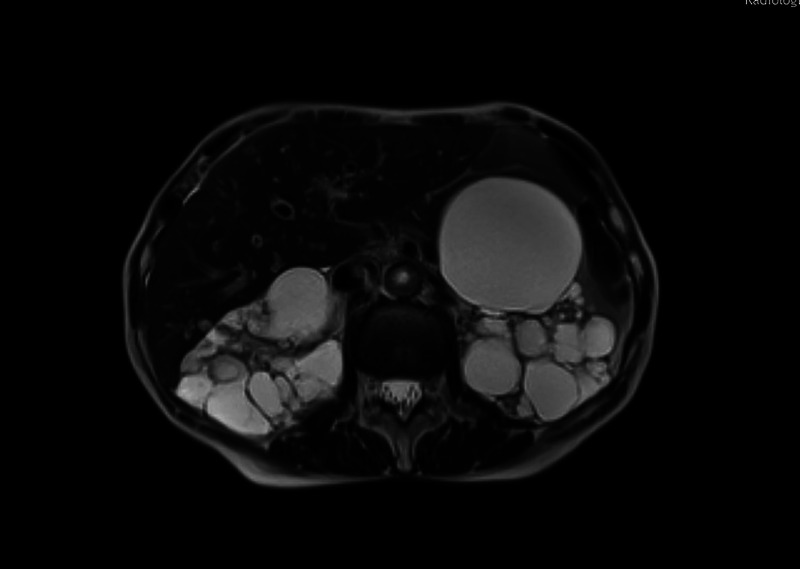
Initial magnetic resonance imaging demonstrating a large cystic lesion adjacent to the pancreas along with polycystic kidney disease.
